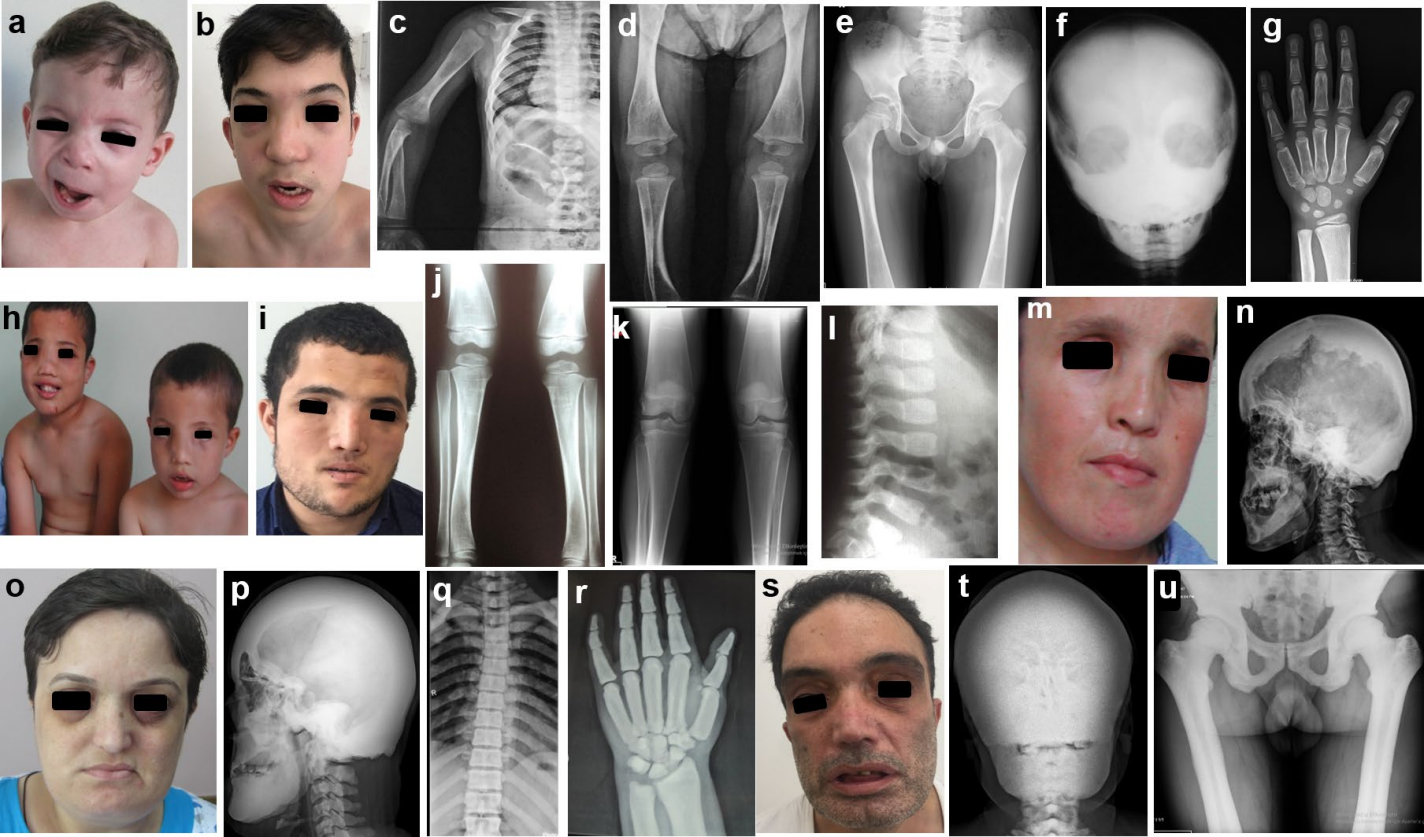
Four patients with craniometaphyseal dysplasia: P1 at the age of 18 months (a, c, d, f), 12 (b) and 8 years (e, g). P2 at the age of 8.5 and 22 years (h, i). P3 at the age of 5.5 (h, j, l) and 16 years (k). Facial palsy and typical facial features including long face, broad nasal bridge, hypertelorism, midface hypoplasia, and prognathism were revealed on photographs (a, b, h, i). P4 had mild dysmorphic face at the age of 44 years (m). Cranial radiographs of P1 (f) and P4 (n) reveal diffusely sclerotic calvarium with obliteration of sinuses. Radiographs of long bones show widening and radiolucency of metaphyses and diaphyseal sclerosis of long bones during early childhood (c, d, j). Note diaphyseal sclerosis has resolved over time (k). No sclerosis on pelvis, hand, and vertebrae graphies (e, g, l). P5 and P6 with sclerosteosis-1, at the age of 37 (o–r) and 45 years (s–u), respectively. Long, flat face, broad forehead, prognathism, and macrocephaly (o, s), diffuse cranial sclerosis (p, t) and significant hyperostosis on the vertebrae, pelvis, and tubular bones (q, r, u)
